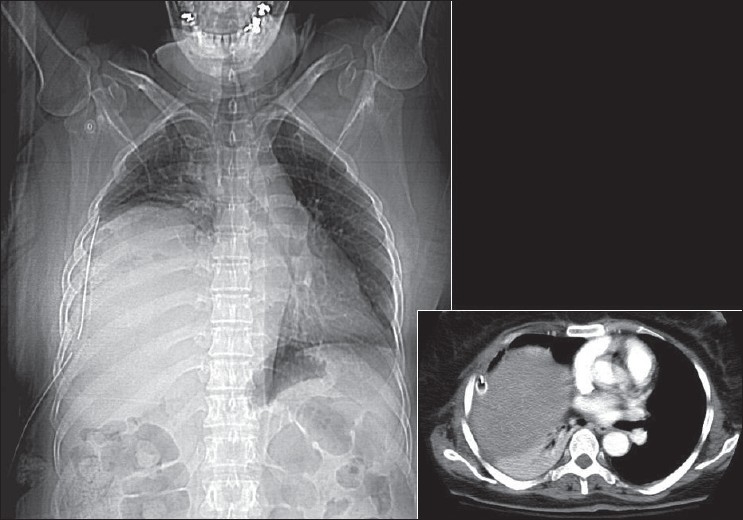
A CT scout film shows a subpulmonic effusion with a misplaced pleural drain. The axial scan confirms the presence of a subpulmonic effusion and depicts the misplaced pleural drain
